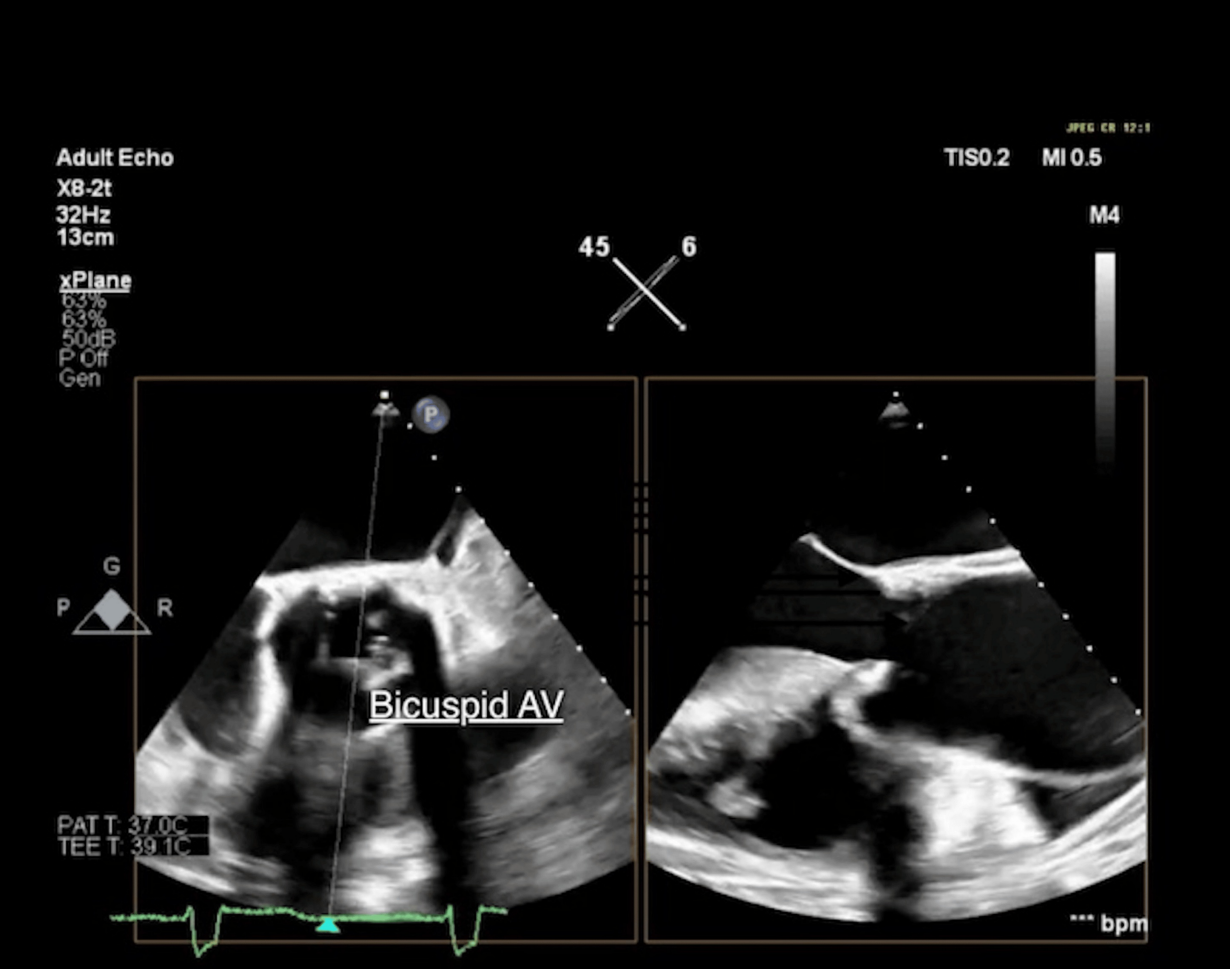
Transesophageal echocardiogram (TEE): Bicuspid aortic valve (AV) in both aortic valve short axis view (Left) and long axis view (Right).
